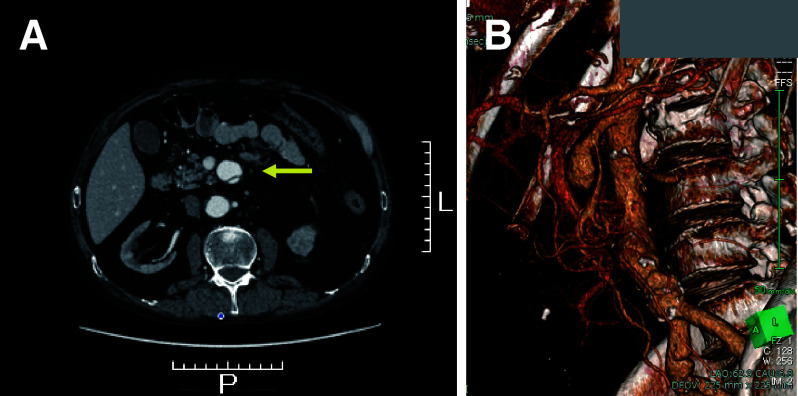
Fig. 1 (A) Preoperative enhanced CT showed a 2.4-cm dissected SMA aneurysm (arrow). (B) Three-dimensional CT. CT: computed tomography; SMA: superior mesenteric artery.
